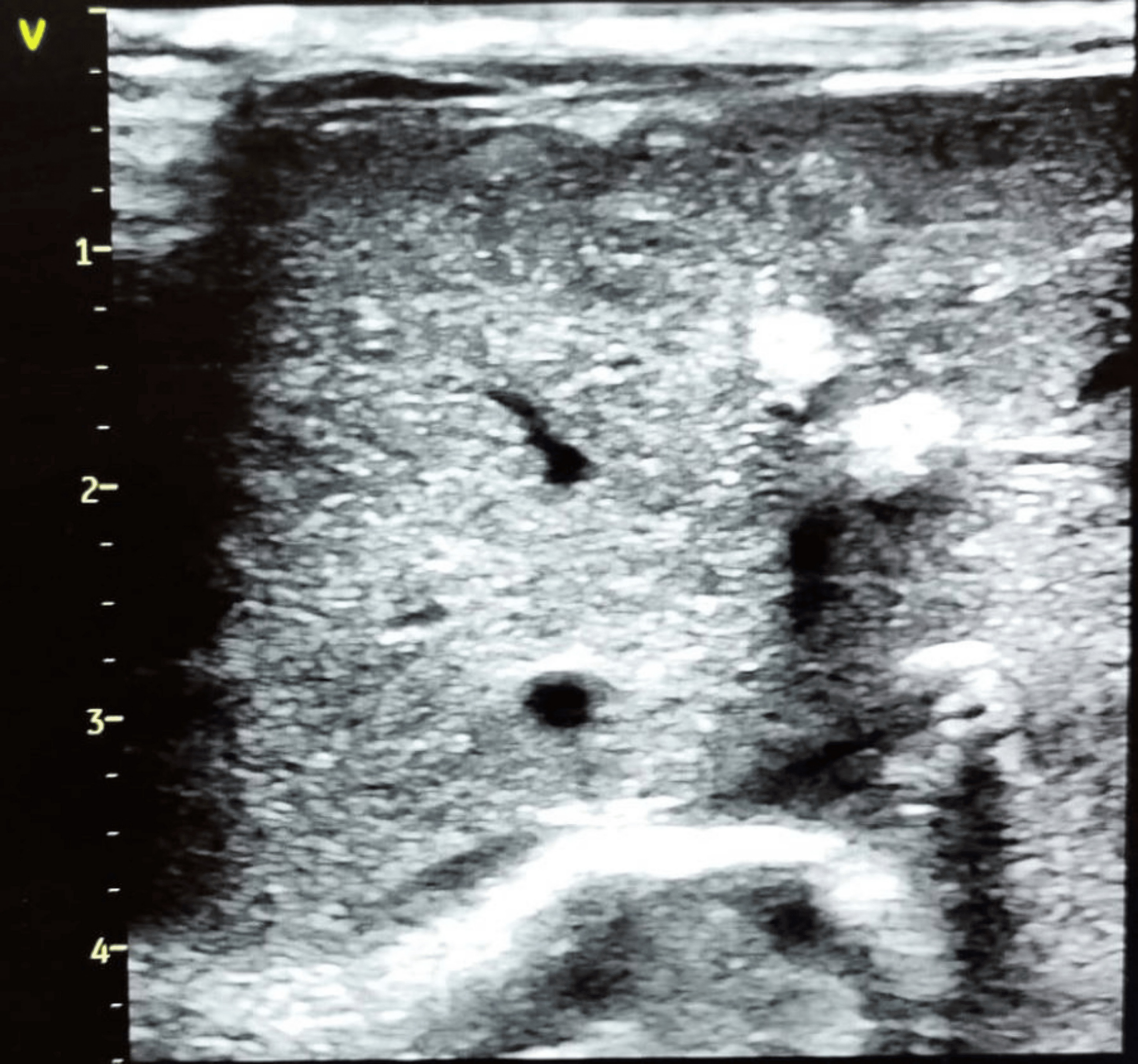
Abdominal ultrasound Abdominal ultrasound showing multiple hyperechoic images consistent with calcifications
